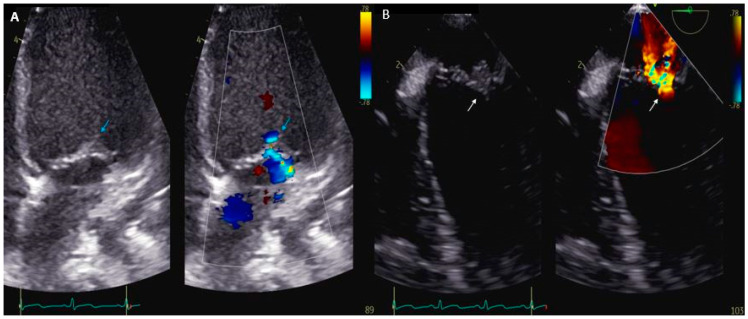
(A) Transthoracic echocardiography of a 5-year-old girl with infective endocarditis of the mitral valve with mild regurgitation (blue arrow). (B) Transesophageal echocardiography of the same patient that enables a better visualization of the vegetation on the anterior leaflet, as well as a better characterization of the regurgitation (white arrow).
